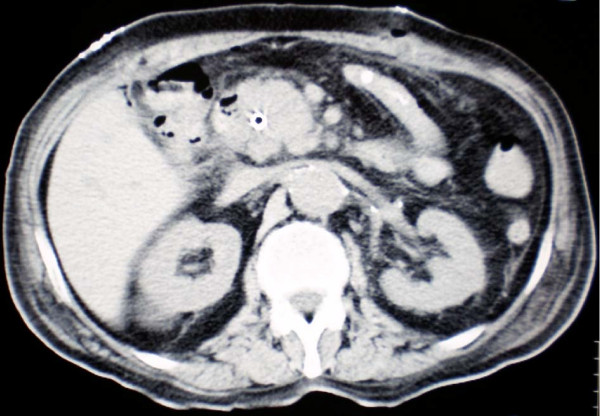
Abdominal CT scan image showing the well circumscribed lesion of the pancreatic head (the common bile duct stent is clearly discerned).
